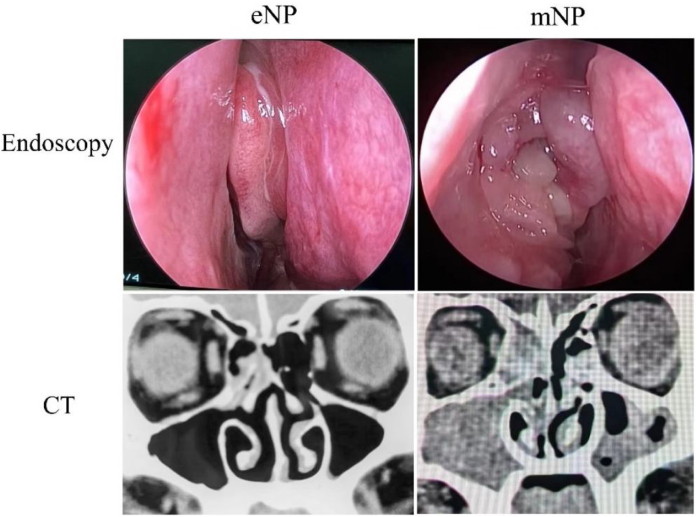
Representative images of eNP and mNP in endoscope and CT scan.
